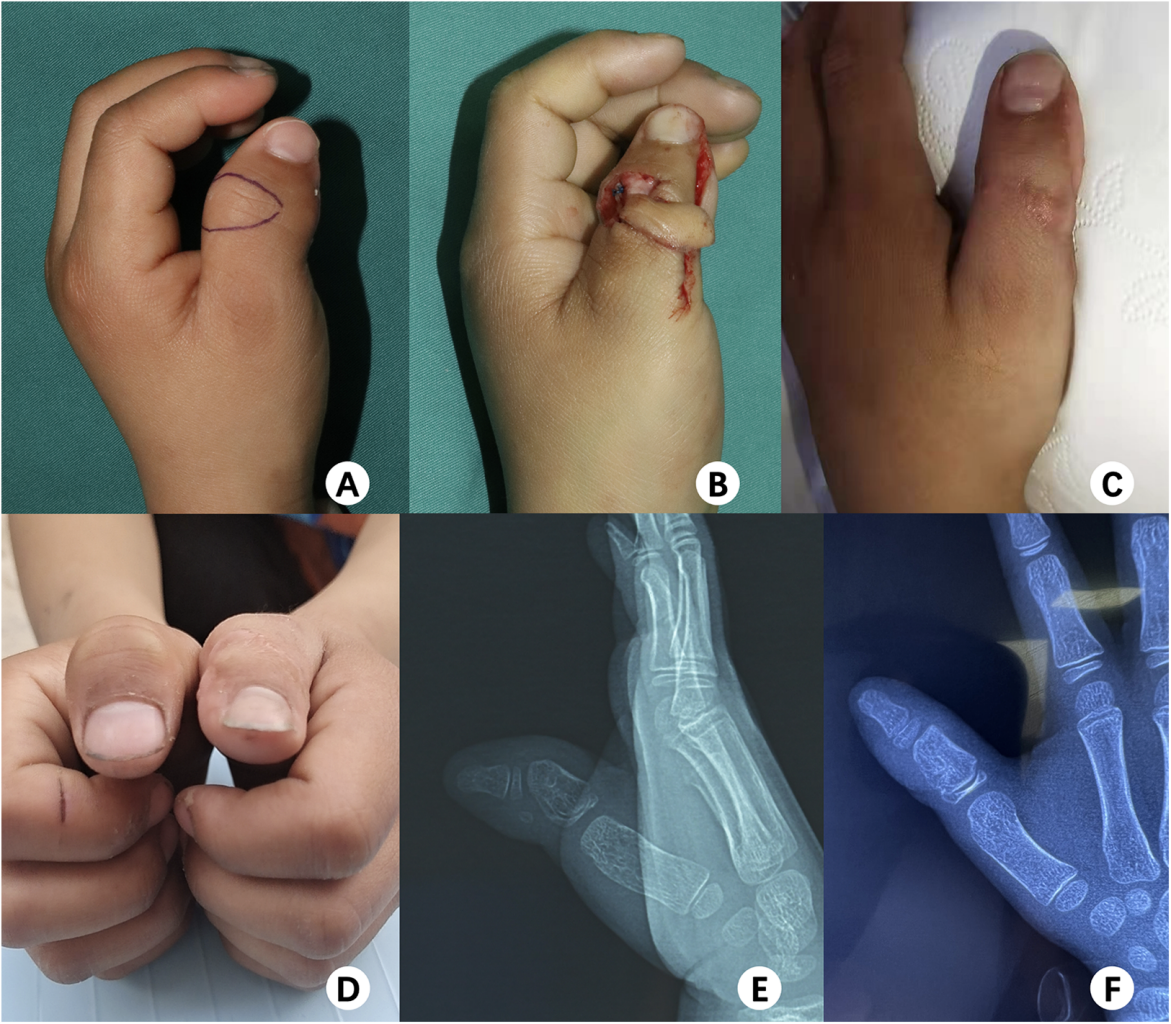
Seven-month follow-up following reconstruction in a 6-year-old boy with deviation of the left thumb. (A) Intraoperative, showing the design of the flap; (B) intraoperative, flap harvested; (C,D) good appearance and excellent function; (E,F) preoperative and postoperative x-rays, respectively.
